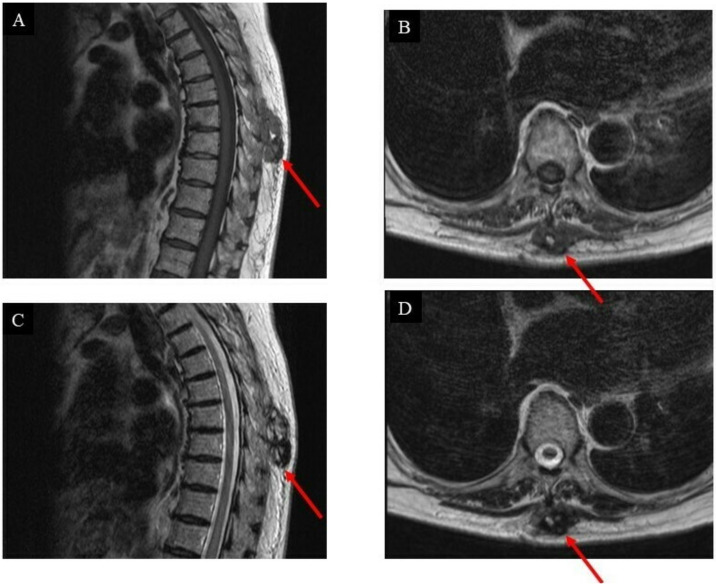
Images of the intrinsic back musculature of a 78-year-old female patient before treatment: (A) sagittal magnetic resonance imaging (MRI) T1-weighted sequence; (B) axial MRI T1-weighted sequence; (C) sagittal MRI T2-weighted sequence; (D) axial MRI T2-weighted sequence.
